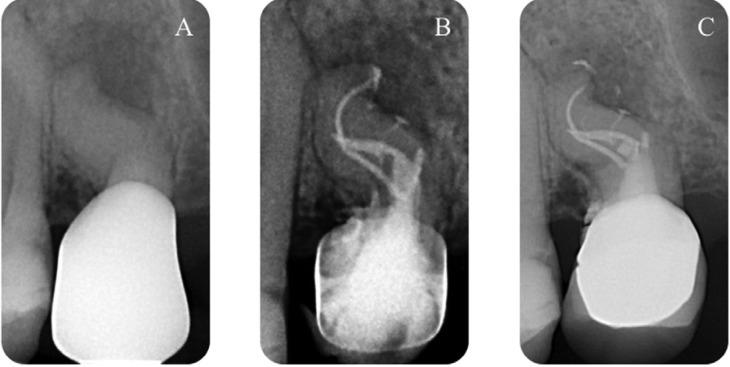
A) Pre-operative radiograph shows one root and an endodontic dilacerated anatomy, B) Post operative radiograph shows two well-obturated canals as well as the lateral canals, C) 1 year later, radiograph shows the healing of periapical lesion.
